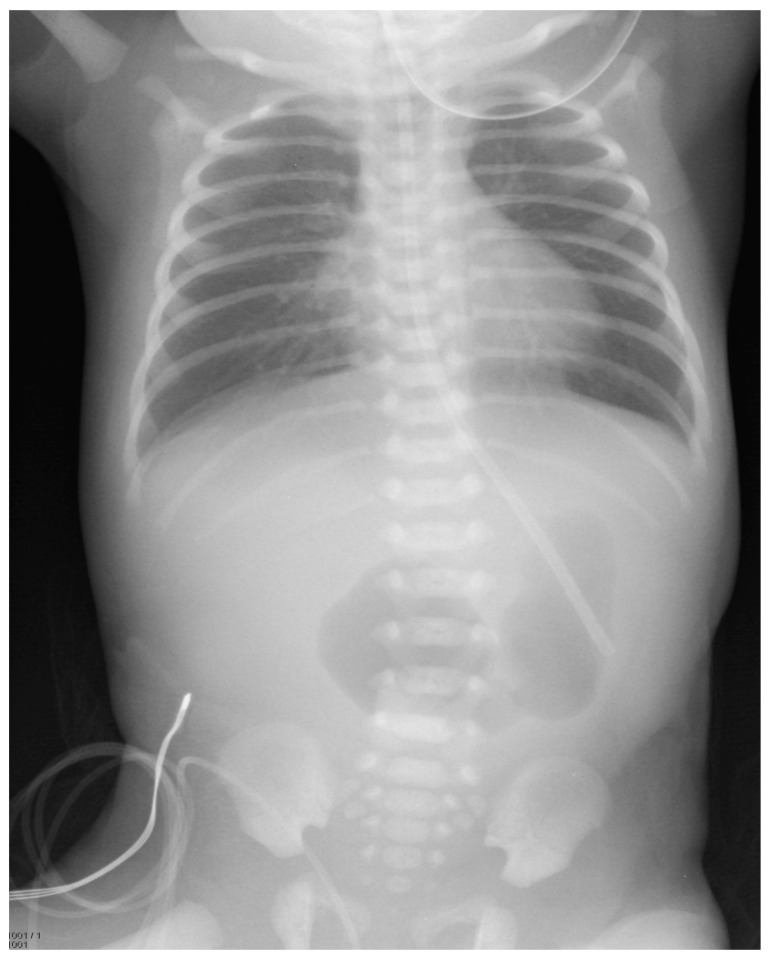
Plain abdominal radiograph taken soon after birth, showing a double-bubble in the lower abdomen.
